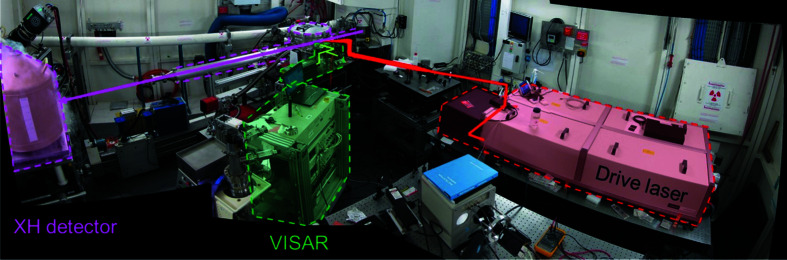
A photograph of the experimental hutch in November 2018. Beams are shown as solid arrows, whereas instruments are shown as dashed lines. X-rays are shown in pink, the drive laser in red and the shock diagnostics in green. For scale, the laser is 2 m long.
